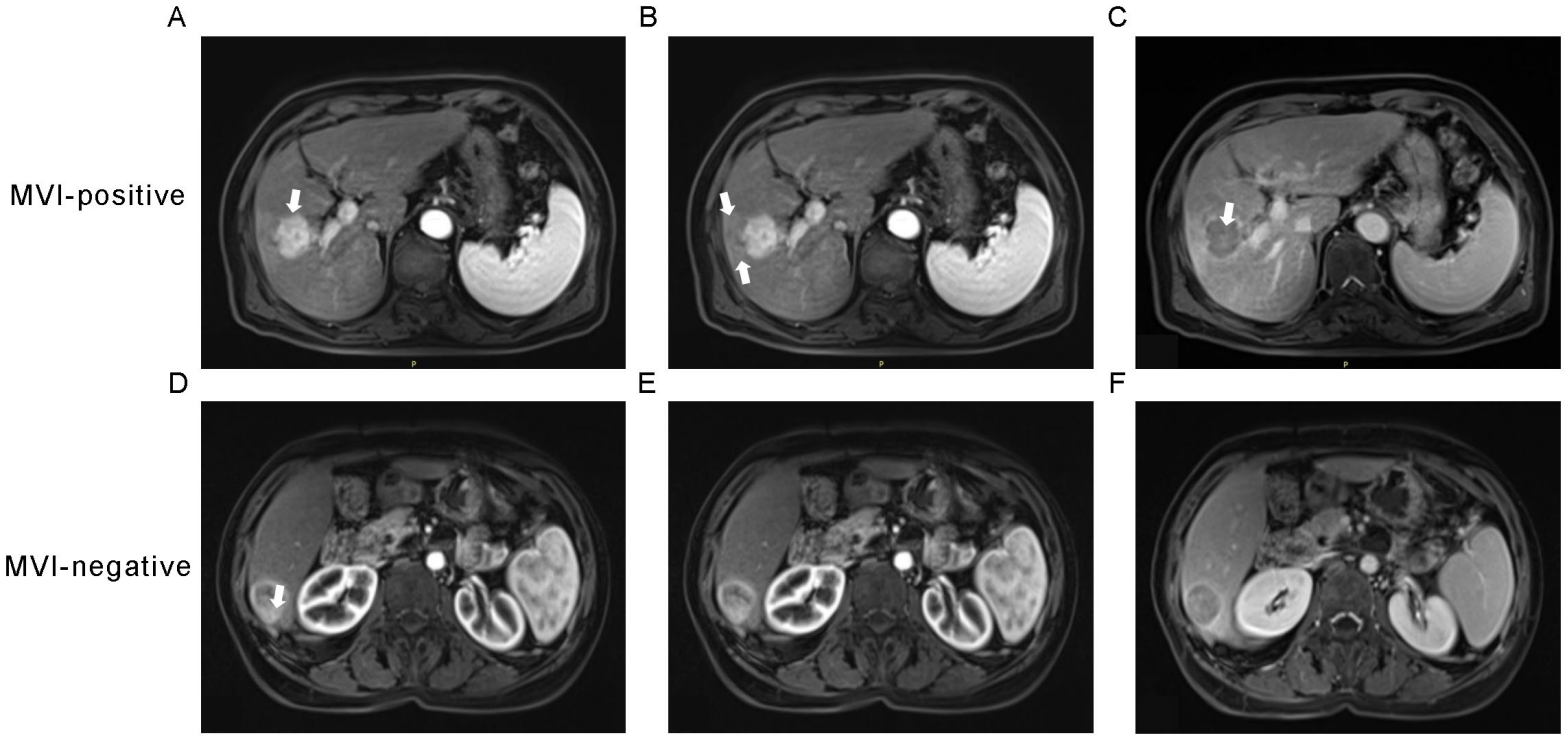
Characteristic MRI images of microvascular invasion (MVI)-positive and MVI-negative hepatocellular carcinoma. (A, B) The axial arterial phase image shows a non-smooth hype vascular tumor (arrow) with peritumoral enhancement (arrows). (C) The delayed phase image exhibits a rapid low signal intensity (arrow) within the tumor. (D, E) The arterial phase image demonstrates a smooth tumor, accompanied by (F) a radiologic capsule in the delayed phase.
